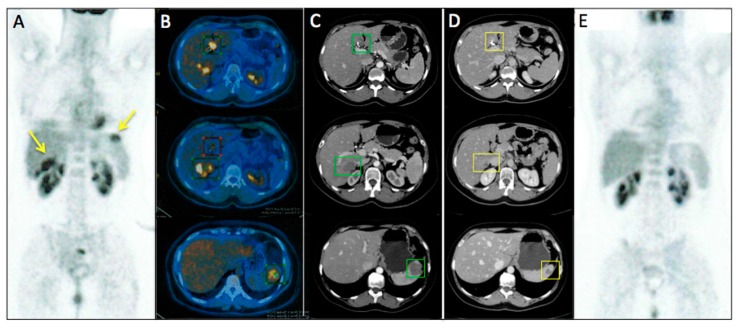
A56-year-old female affected by ovarian cancer with liver and spleen metastasis, already treated with 12 cycles of Bevacizumab-based chemotherapy and 24 hyperthermia sessions on the abdomen, as first-line. Baseline MDCT (C) showed metastasis in III and IV segment of the liver (maximum diameter: 77 mm, green squares) and in the upper spleen (maximum diameter: 61 mm, green square). Baseline whole body ^18^F-FDG PET/CT (A) confirmed liver and spleen involvement by the increased ^18^F-FDG uptake (yellow arrows) detectable also on axial fused PET/CT images (B) in the same sites (green and red squares). Timepoint-2 MDCT (D) evaluation demonstrated significant size decrease of liver and spleen metastasis (yellow squares) with no evidence of ^18^F-FDG uptake on whole body PET/CT (E). According to mRECIST, patient was classified as CR. Ca125 value evaluated at baseline was 790 UI/mL; while Ca125 value evaluated at timepoint-2 was 12 UI/mL. Side effects reported were limited to asthenia and high blood pressure.
